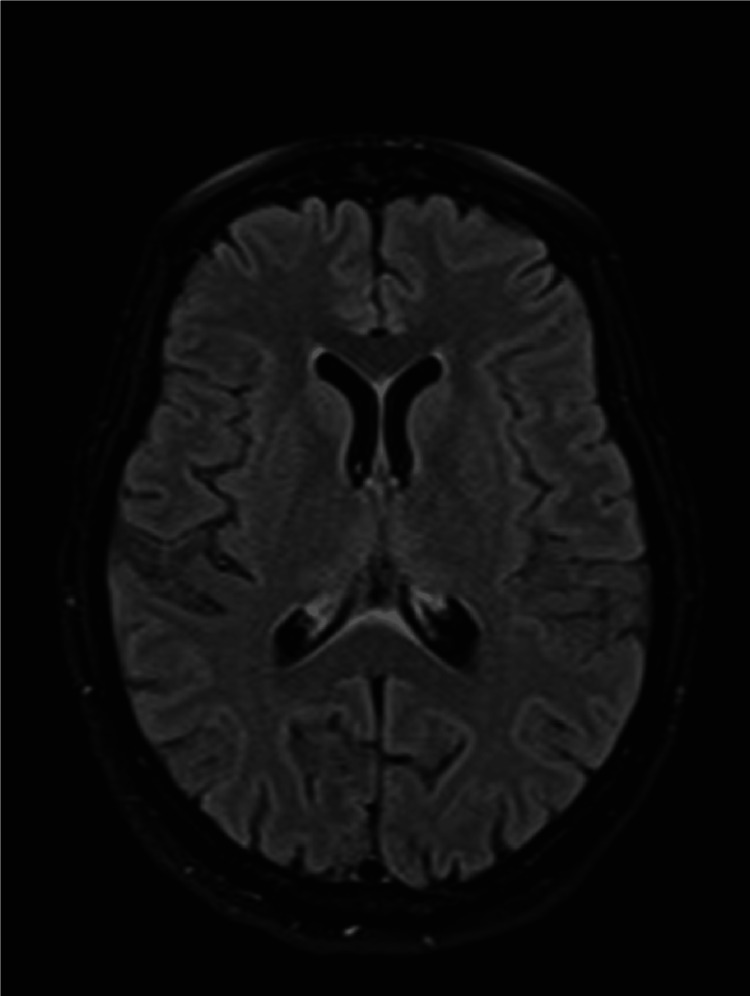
Brain MRI Bilateral symmetric abnormal increased signal intensity on T2/FLAIR sequences in various brain regions, including the mammillary bodies, dorsomedial thalami, tectal plate of the midbrain, periaqueductal grey matter, dorsal aspect of medulla oblongata, and minimally around the third ventricle. Similar signal changes are seen in bilateral frontal cortical grey matter and the central area of the pons with peripheral sparing; restricted diffusivity in specific brain regions; T1 hypointense abnormality; post-contrast enhancement in specific brain regions; basal ganglia and corpus callosum appeared normal; bilateral frontal nonspecific T2/FLAIR high signal spots within white matter; no intracranial collections or abnormal meningeal enhancement observed.
